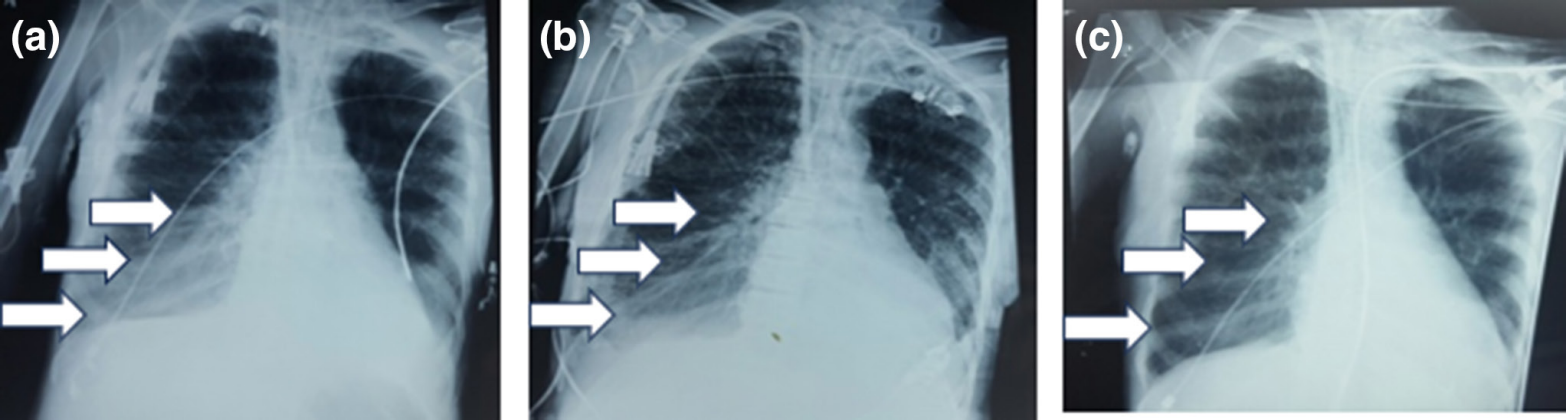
Chest X-ray (CXR) AP view of ventilator-associated pneumonia (VAP) (a) Arrow showing right lower zone dense patch of VAP on the eighty-first day of illness (DOI-81). Panel (b) and (c) showing resolution at (DOI-87) and (DOI-93) respectively.
